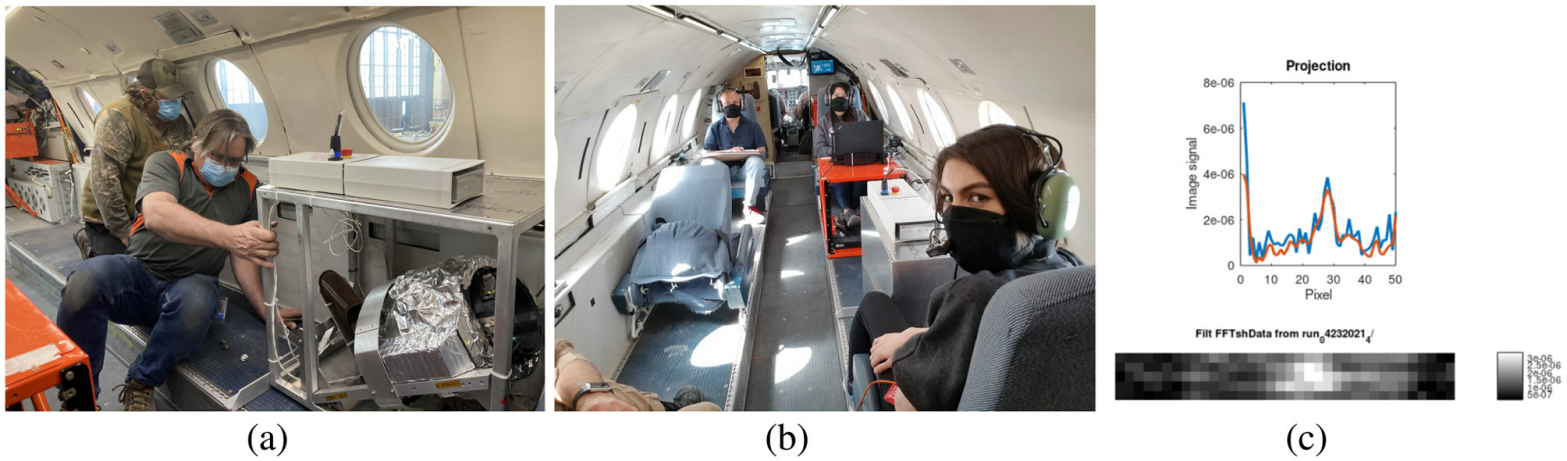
(A) The *Merlin MRI* being installed on the Falcon 20 jet. Here the cover is removed for installation. (B) Flight crew ready for zero-g. The woman in the foreground has her leg in the MRI for imaging during the 20 seconds of each zero-g parabola. (C) Example projection data of a volunteer's lower leg, obtained during zero-g. The projection in the top graph is from the middle natural slice. The image shows stacked projections from the corresponding axial natural slices (isomagnetic surfaces).
